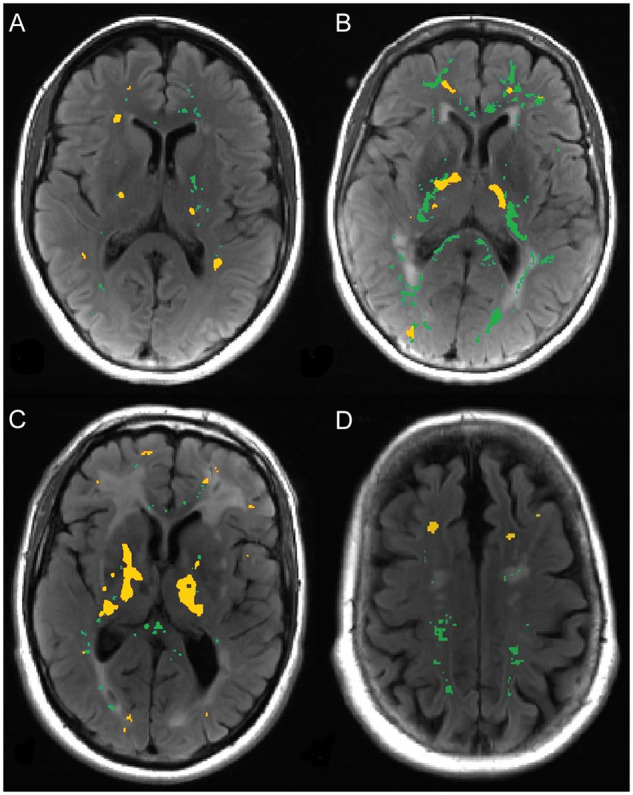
Hotspots of increased BBB permeability and ^11^C-PK11195 binding. Example images from four different SVD subjects (A–D) showing hotspots of increased BBB permeability (green) and ^11^C-PK11195 binding (yellow) overlaid onto T_2_ FLAIR images.
